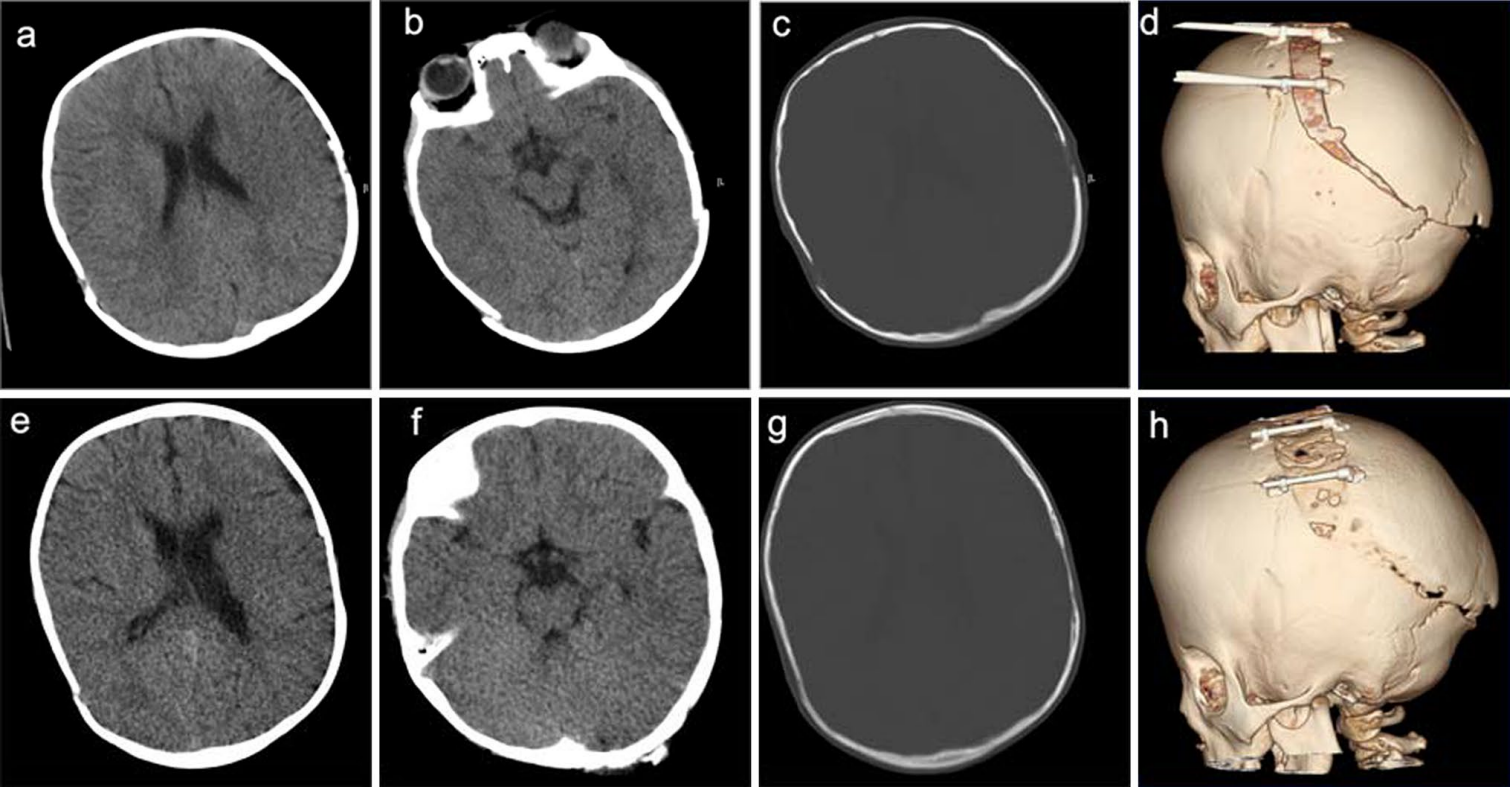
Example of comparison: four categories of view-points in the half-dose method (HDM) and full-dose method (FDM). A 4-year-old female with coronal suture craniosynostosis. Cranial computed tomography (CT) image obtained soon after cranioplasty by the FDM (a–d), and follow-up CT imaged obtained using the HDM at 4 months after the previous CT (e–h). FDM scan parameters: tube voltage, 120 kV; tube current, 220 mA; CTDI-vol, 24.4 mGy; DLP, 589.1 mGy・cm. HDM scan parameters: tube voltage, 120 kV; tube current, 130 mA; CTDI-vol, 12.5 mGy; DLP, 257.8 mGy・cm. In terms of image quality, the brain parenchyma on images obtained by the HDM (e) was relatively coarse as compared to that on images obtained by the FDM (a). However, the corticomedullary boundary of the cerebral hemisphere can be recognized on the HDM image (e). Additionally, the ventricular system (a, e), and 2D (c, g) and 3D (d, h) cranium images seemed to have a similar image quality in HDM and FDM images, without loss of diagnostic value
